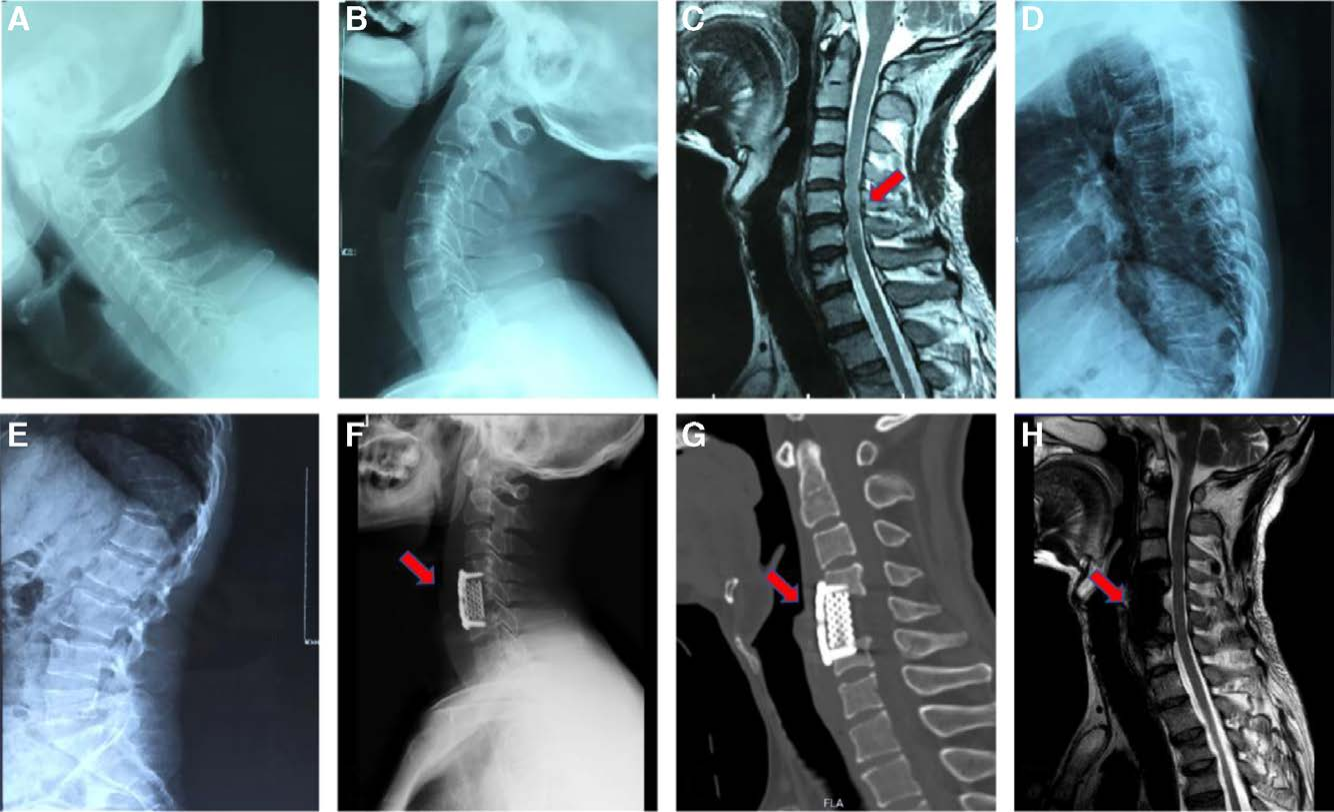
Pre- and postoperative imaging findings of a 44-year-old man who underwent cervical discectomy for cervical disc herniation and was later identified as having adefovir dipivoxil-induced Fanconi syndrome associated with hypophosphatemic osteomalacia. (A, B) Preoperative lateral radiographs of the cervical spine in extension and flexion positions. (C) Sagittal MR image showing disc herniations at C4/5, C5/6, and C6/7 (red arrow), with corresponding spinal cord compression. (D and E) Lateral radiographs of the thoracic and lumbar spine demonstrating multiple flattened vertebral bodies with biconcave changes, suggesting osteomalacia. (F–H) Postoperative follow-up images. (F) Lateral cervical radiograph, (G) CT scan, and (H) sagittal MR image confirming stable internal fixation (red arrows) and satisfactory spinal cord decompression.
